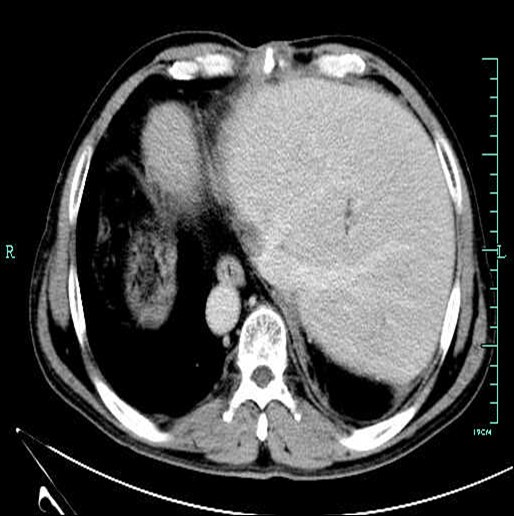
Graft image surveillance at 3 months post transplant. Abdominal computed tomography scan shows a normal liver graft in the up left quadrant.
